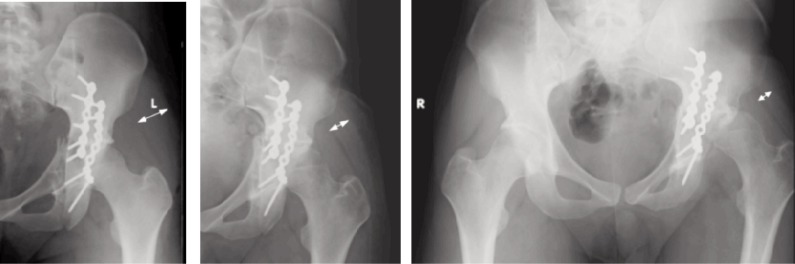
Serial radiographs of Case One after surgery, at 2 months and at 6 months, showing progressive wasting of the gluteus medius (white arrows), and eventual subluxation of the left hip.
